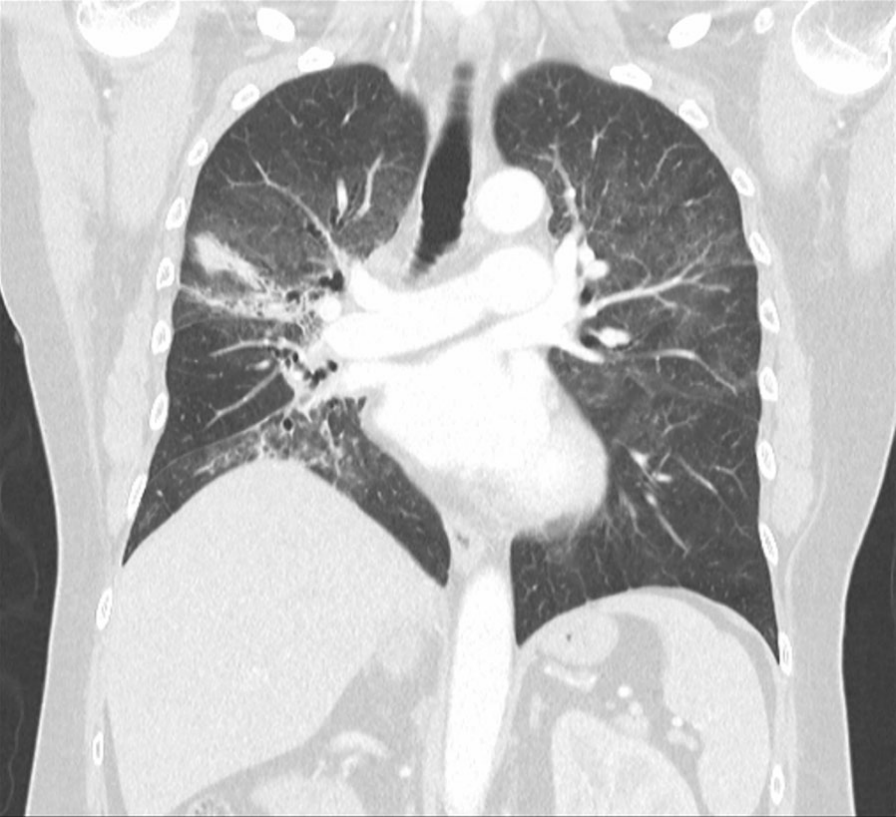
Case 1—CT chest 2 months after commencement of pneumonitis treatment with incomplete resolution of pneumonitis changes
